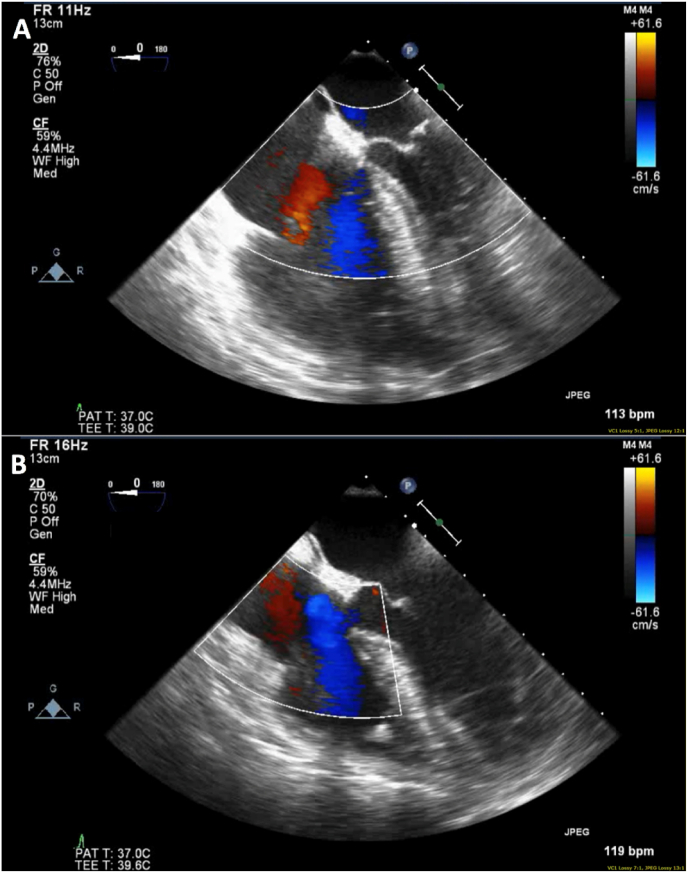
Intraoperative transesophageal echocardiogram images showing a significantly dilated right ventricle (Panel A) that normalized (Panel B) after surgical embolectomy.
